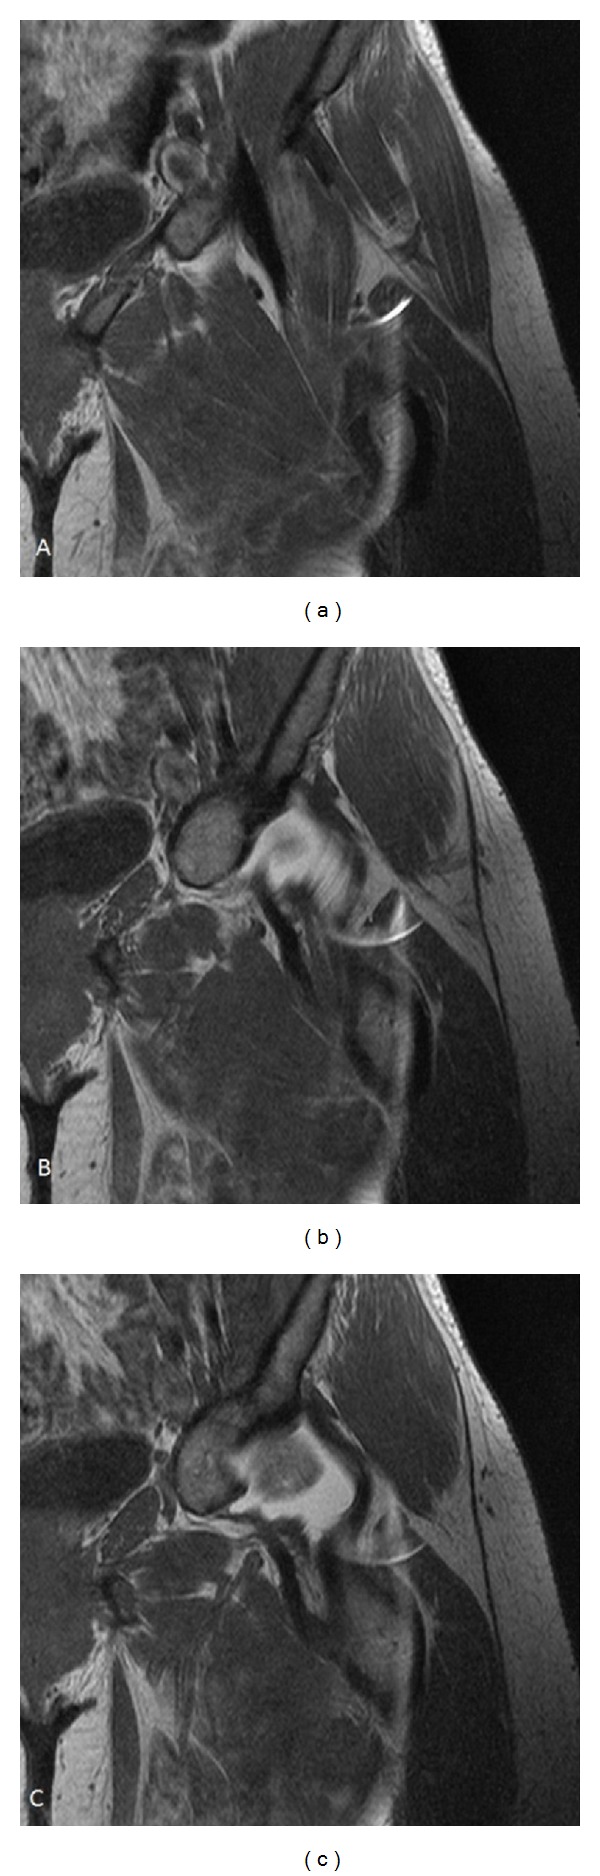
A series of T2-weighted, coronal MR arthrograms of the left hip taken 3 months prior to the patient's repeat left psoas release. The images identify an iliopsoas pseudo-tendon anterior to the hip joint (a). This pseudo-tendon extends beyond the hip joint (b) and is inserted into the superior aspect of the lesser trochanter (c).
